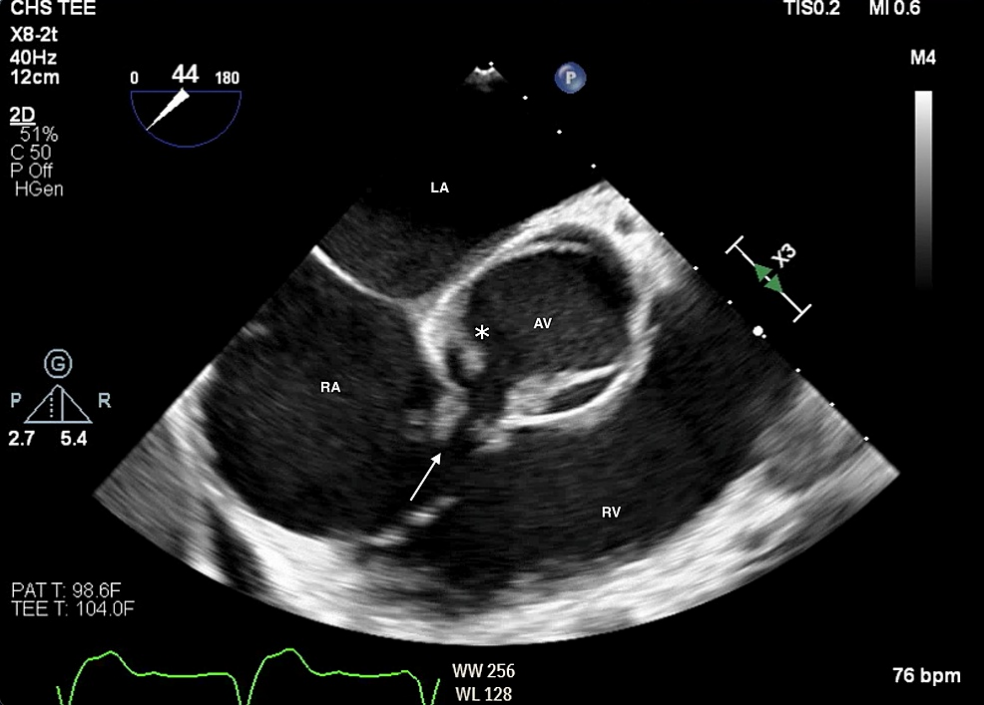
Trans-esophageal echocardiography, mid-esophageal short-axis view Demonstrates a bicuspid aortic valve (AV) with perforated leaflet (asterisk) and ruptured aortic root into right atrium (RA) forming Gerbode defect (arrow). LA: left atrium, RV: right ventricle.
